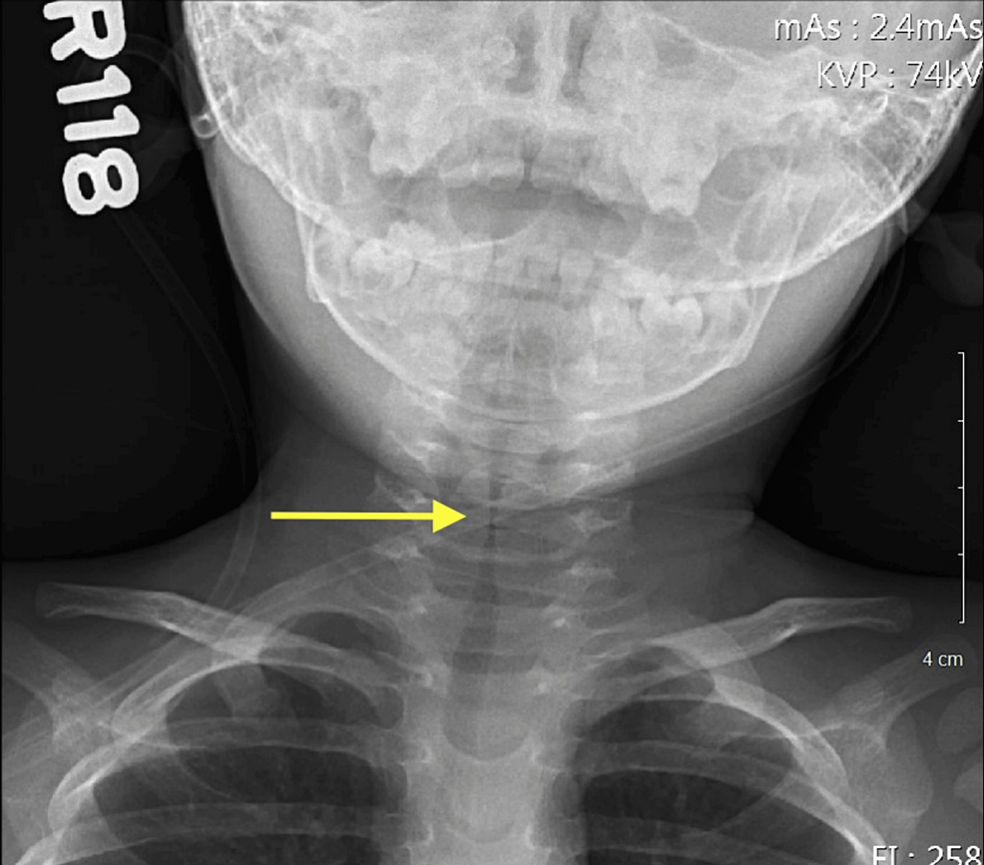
Posterior-anterior radiograph showing recurrent subglottic stenosis and diffuse tracheal narrowing at second pediatric intensive care unit (PICU) admission (one day after discharge from Initial PICU)
